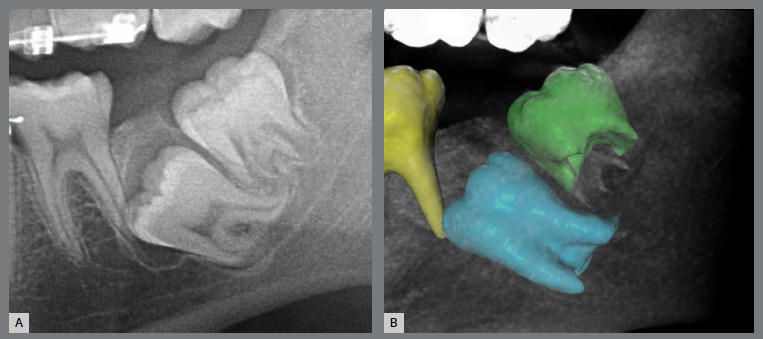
Images of impaction of the mandibular left second molar: A)panoramic radiograph close-up; and B) cone-beam computed tomography close-up.
